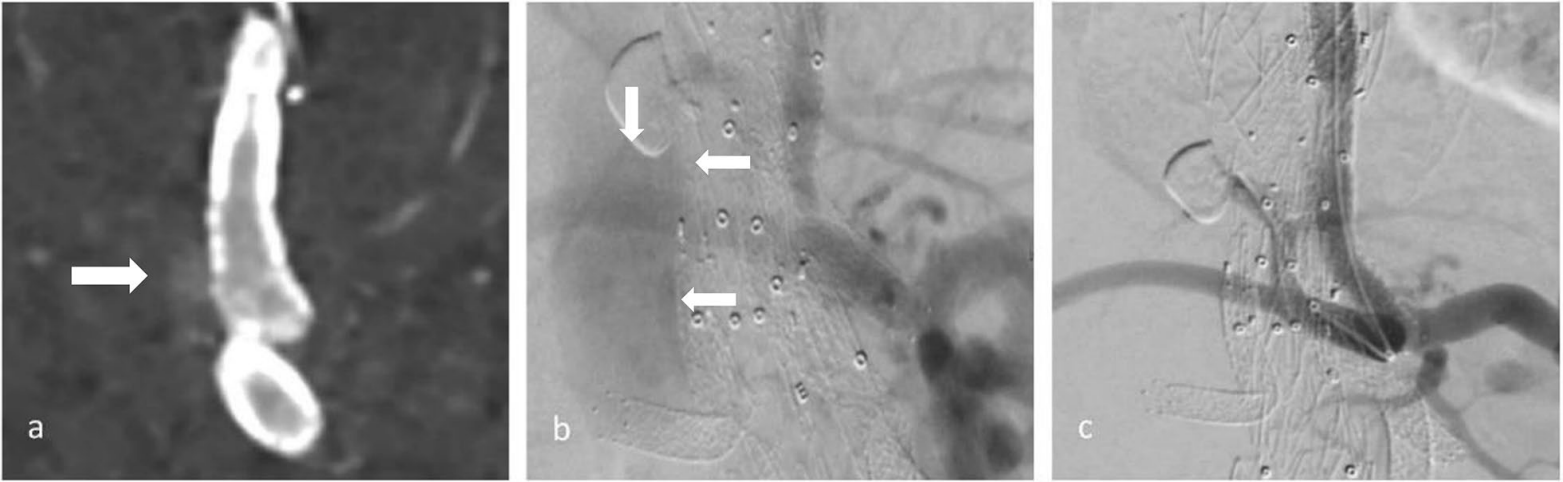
Membrane disruption of BSG in celiac trunk with visible endoleak in CTA (a) and angiography before (b) and after religning (c)
